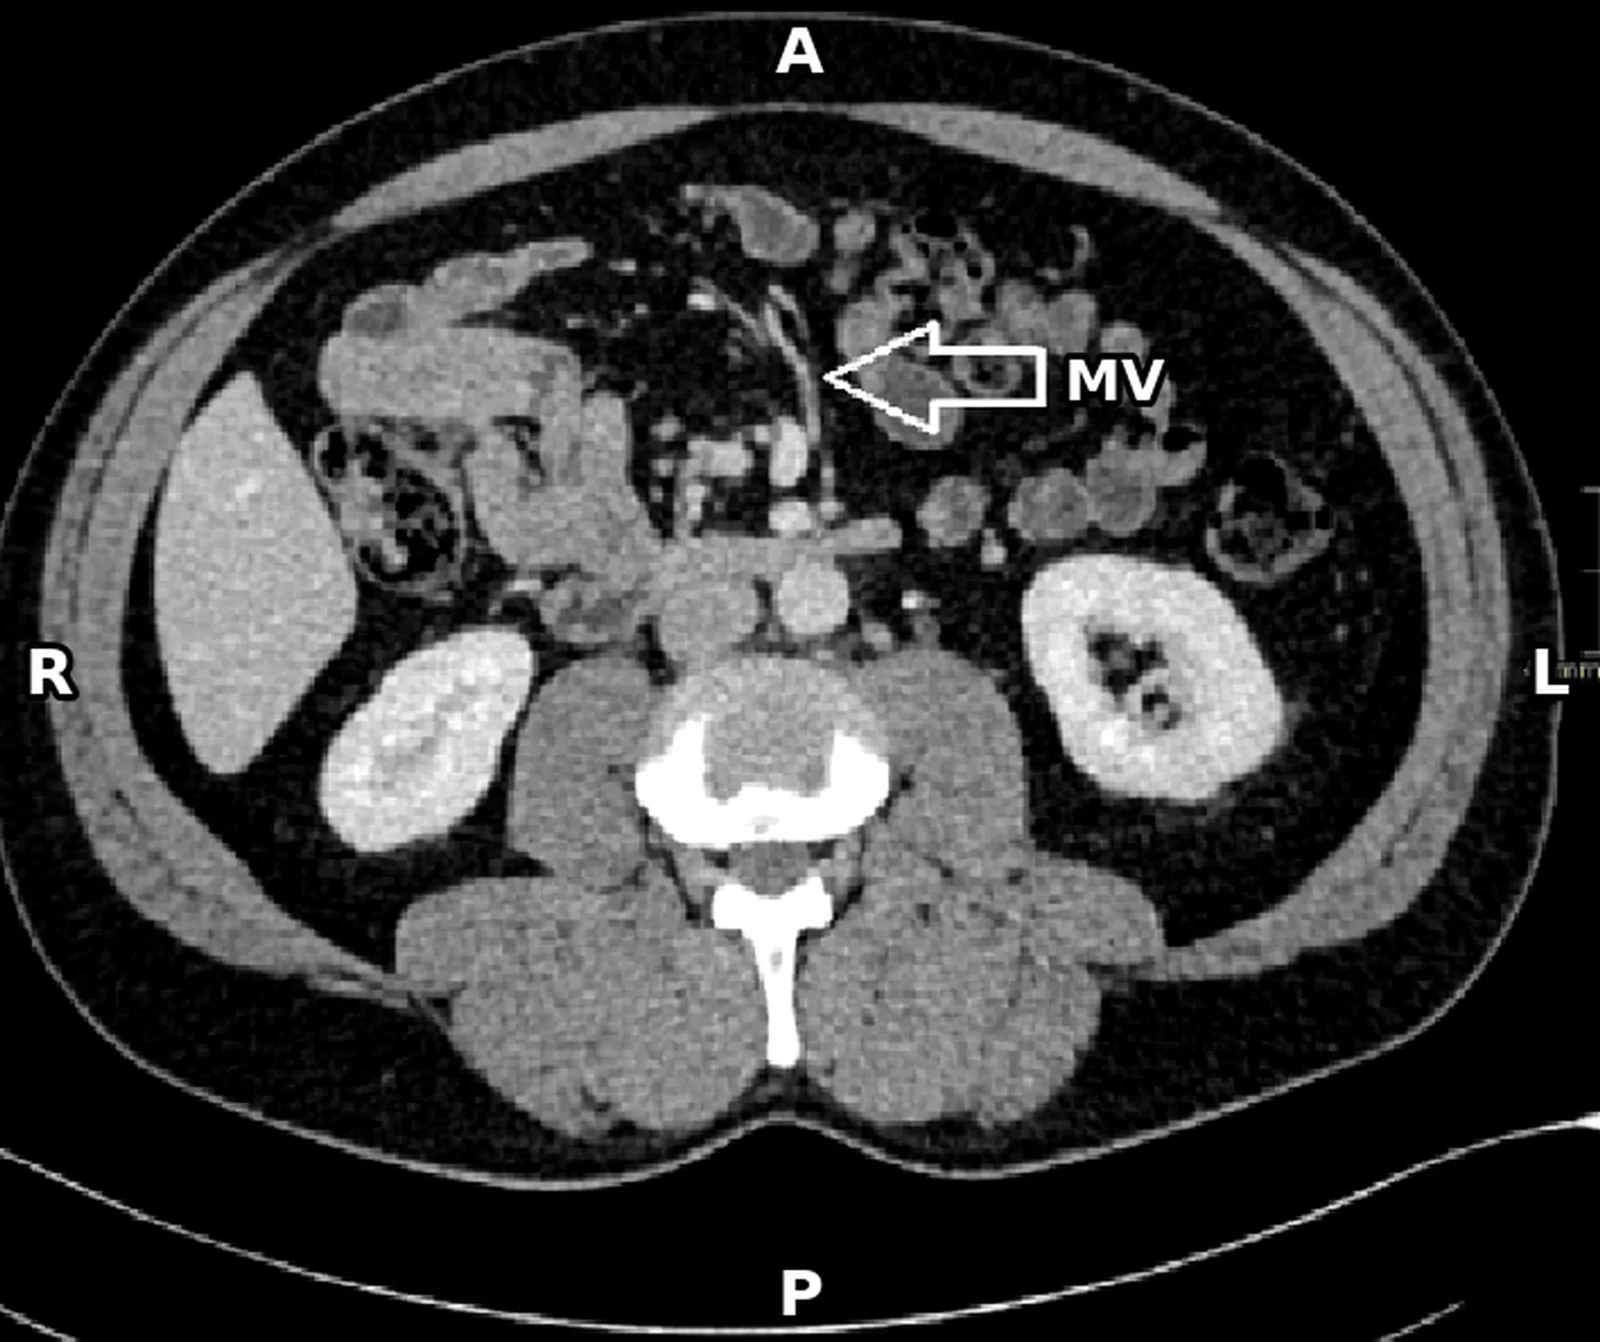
Axial contrast-enhanced CT image of patient 2. The white arrow labeled MV indicates the spiral configuration of the mesenteric vessels. R, L, A, and P indicate the patient’s right, left, anterior, and posterior sides, respectively. CT: computed tomography
